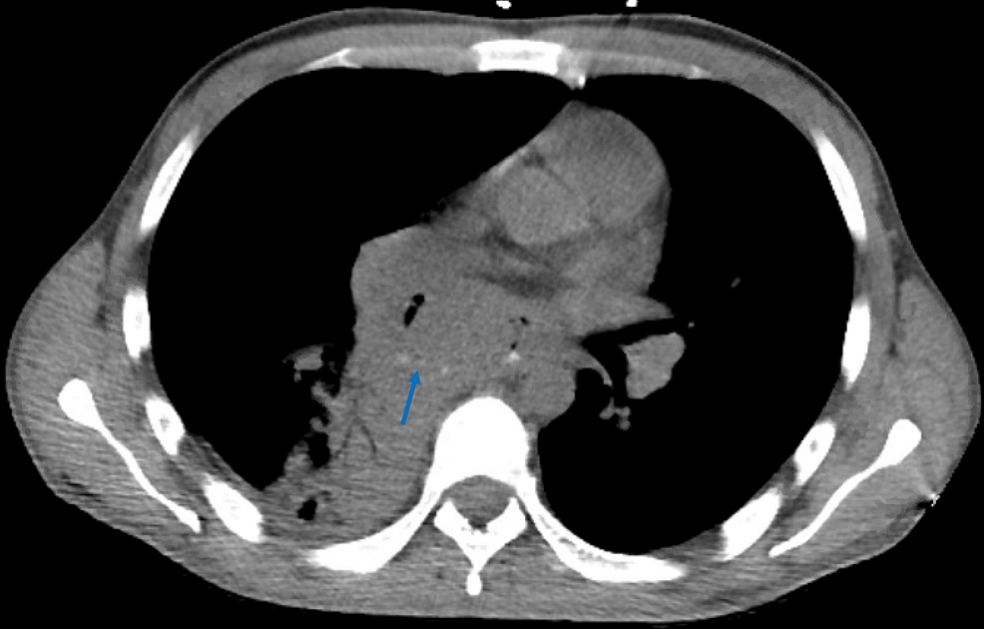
CT scan showing contrast extravasation (blue arrow) into mediastinal mass (1.42x).
